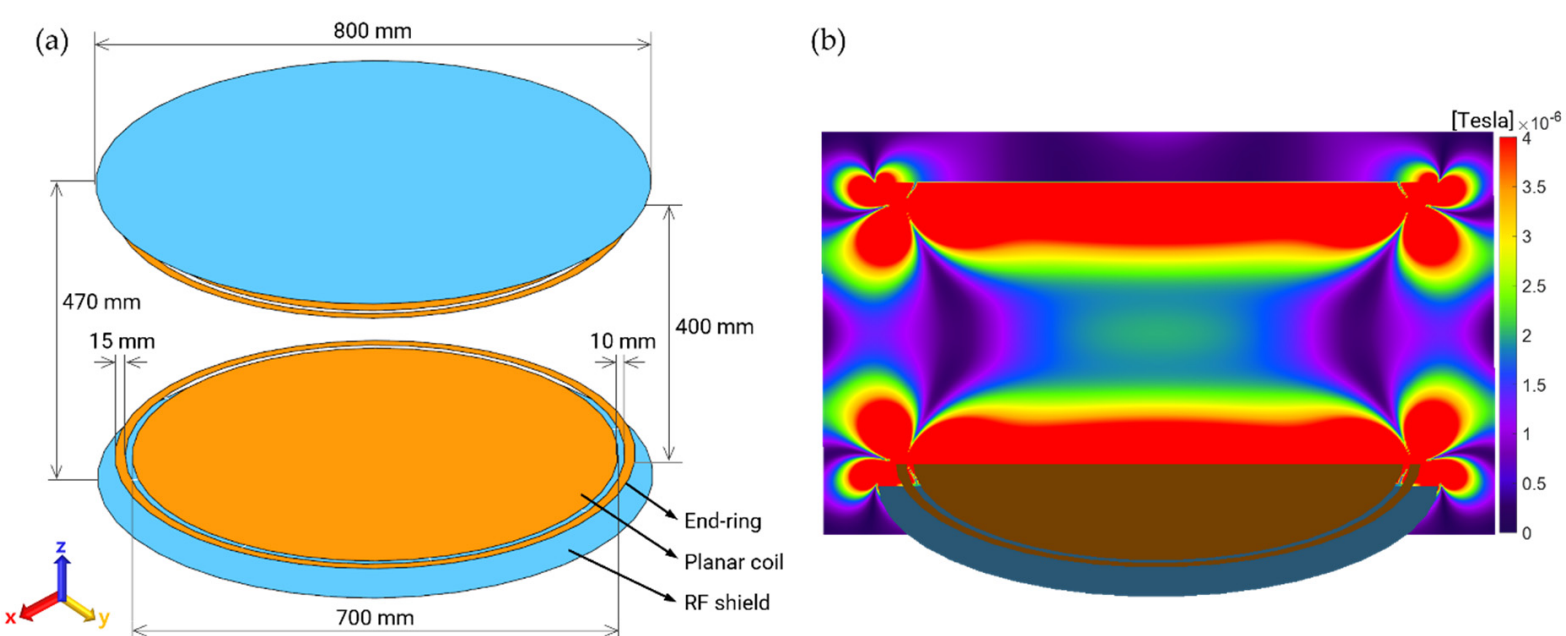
(a) Numerical modeling of the planar T_X_ RF coil. The electromagnetic field generating the MR imaging signal forms in between two plana coil elements; (b) Excitation magnetic field (B_1_^+^). The applied RF power was scaled to create 2 μT of B_1_^+^ at the center of magnet (upper elements of Tx RF coil were removed for better visualization).
